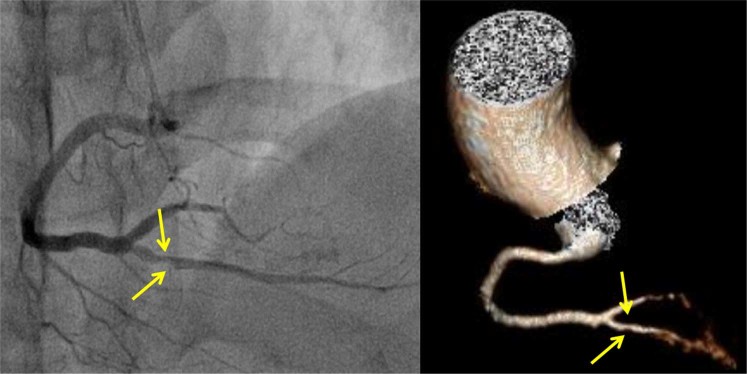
False negative finding. Right posterior descending artery stenosis, detected via invasive coronary angiography (a), and underestimated (false negative) via coronary computed tomographic angiography (b) under 100 kVp/150 mAs in a 66-year-old woman (effective radiation dose, 1.32 mSv; BMI, 24.0 kg/m^2^, mean heart rate, 58 bpm).
